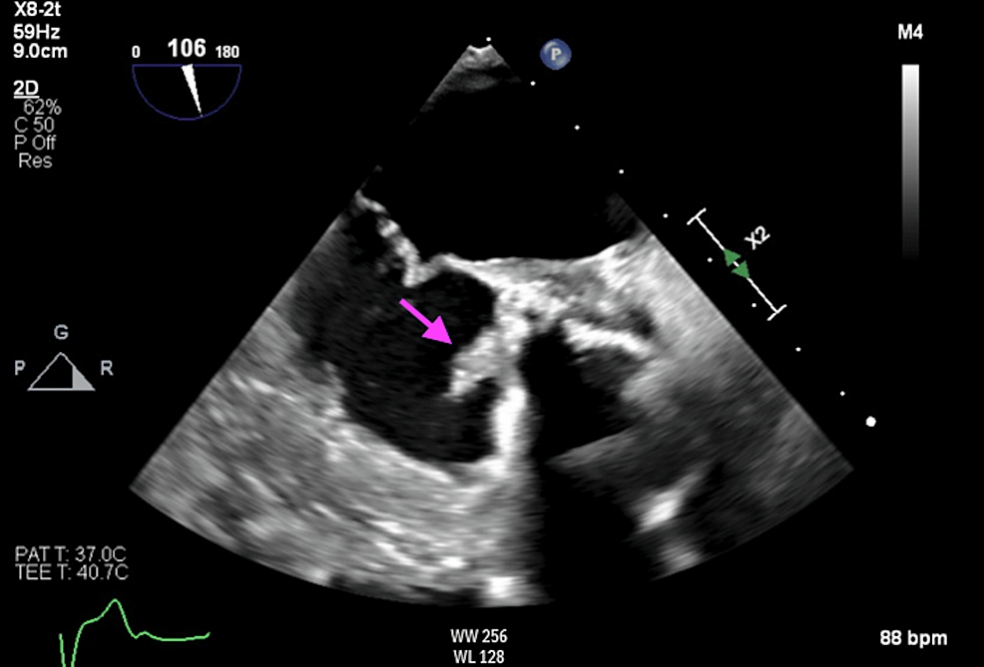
Transesophageal echocardiogram mid-esophageal view Arrow indicating aortic root thickening and irregular vegetation on the ventricular aspect of the posterior aortic valve ring extending to the aortomitral continuity, consistent with a para-valvular abscess.
